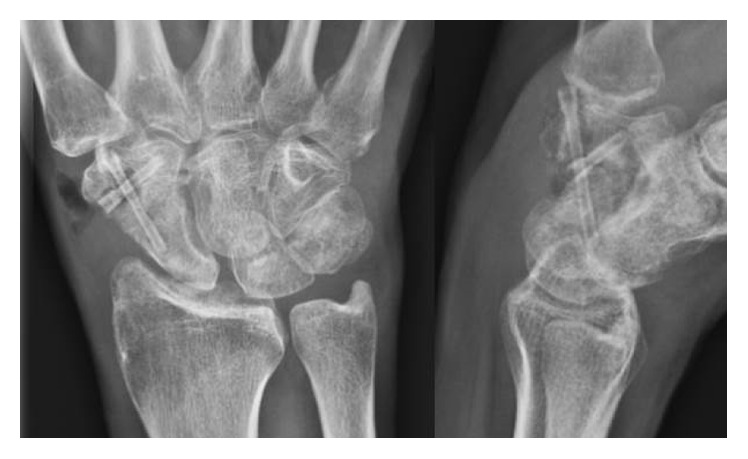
X-ray of the same wrist as in Figure 3, 6 weeks after arthrodesis showing loosening and backing out of both screws.
